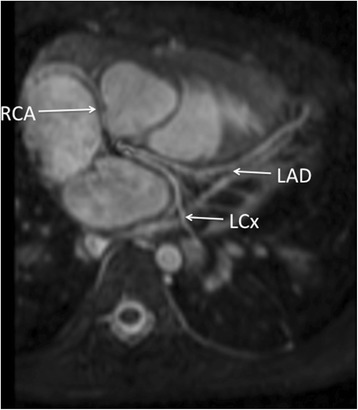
8-year old boy with ventriculo-arterial discordance showing single coronary artery. Echocardiography was not able to demonstrate this
